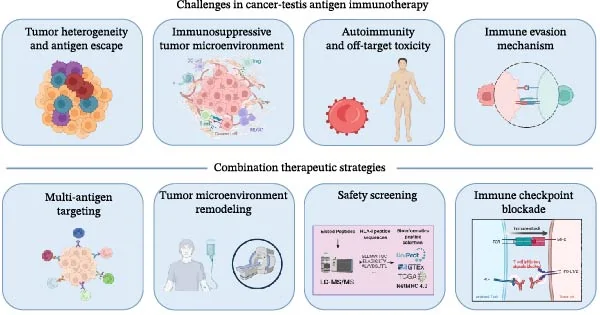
Challenges and combination strategies in cancer‐testis antigen (CTA)‐based immunotherapy.
